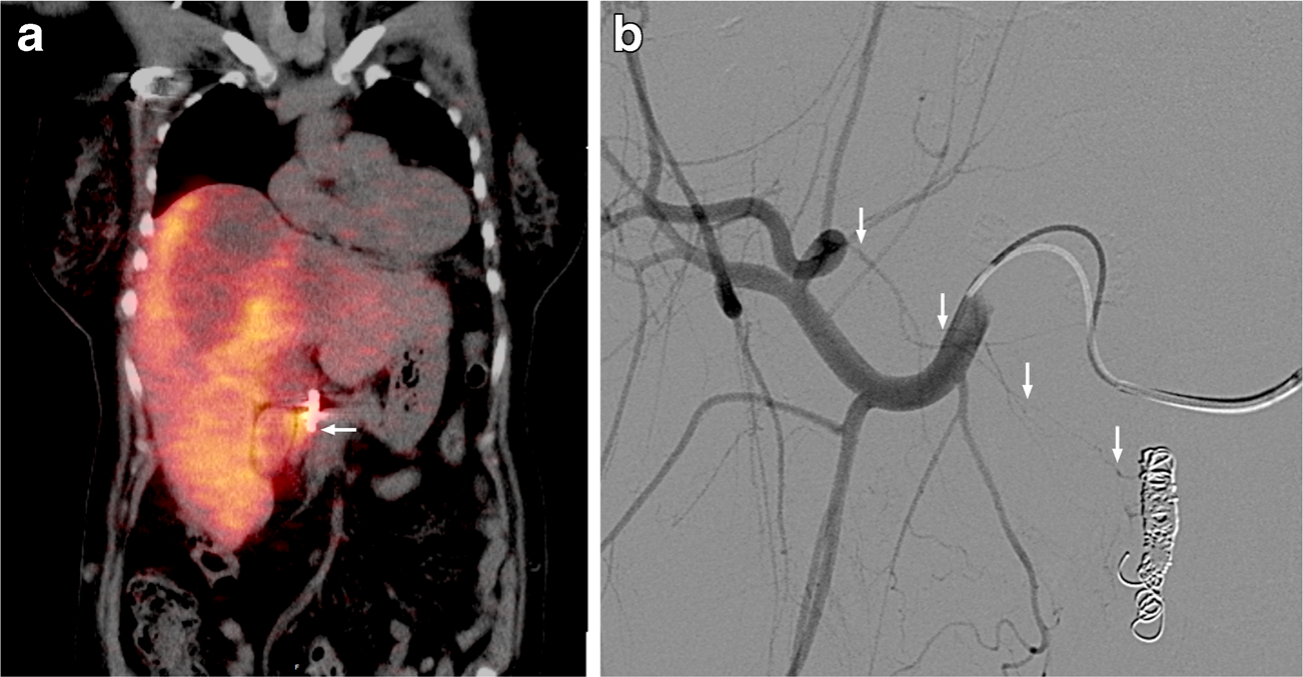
A 62-year-old female with liver metastases of a pancreatic adenocarcinoma. (a) SPECT/CT after administration of ^166^Ho scout dose. Extrahepatic deposition in the duodenum (arrow). (b) Digital subtraction angiography shows flow redistribution in intrahepatic collateral (arrows), directly following coil embolization of gastroduodenal artery. Development of new hepatico-enteric collaterals after previous coil embolization has been described before [15]
